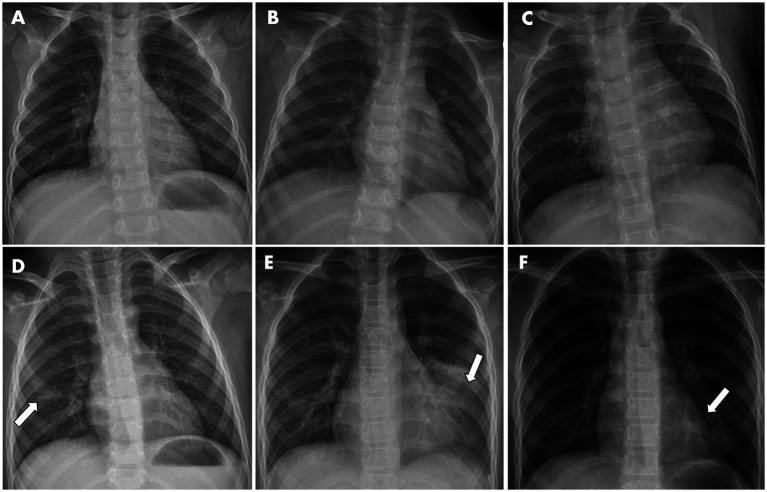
Representative X-ray graphs of pediatric inpatients infected with IAV, taken upon hospital admission, showed typical pathological changes in the lungs, without (A–C) or with (D–F) further pneumonia development. (A) From a 3-year-old girl with fever and cough symptoms. X-ray image showed the texture of both lungs was increased and slightly blurred. (B) From a 2-year-old girl having cough and fever. (C) From a 2-year-old boy with fever. Images showed the texture of both lungs was increased and slightly blurred, but no obvious exudative lesions were observed. (D) From a 6-year-old boy with fever. Image showed patchy faint shadow with blurred margins in the right lower lung region (indicated by arrow). (E) From a 9-year-old boy having cough and fever. Image showed a flocculent high-density shadow with blurred margins in the left middle and lower lung region (indicated by arrow). The morphology and size of the cardiac shadow were not abnormal, and the right rib-diaphragm angle was sharp with a smooth diaphragmatic surface. The left rib-diaphragm angle was shallow and obtuse. (F) From an 8-year-old girl with fever. Image showed a flocculent high-density shadow with blurred margins was observed in the left lower lung region (indicated by arrow), but no obvious exudation or solid changes in both lungs were noticed.
